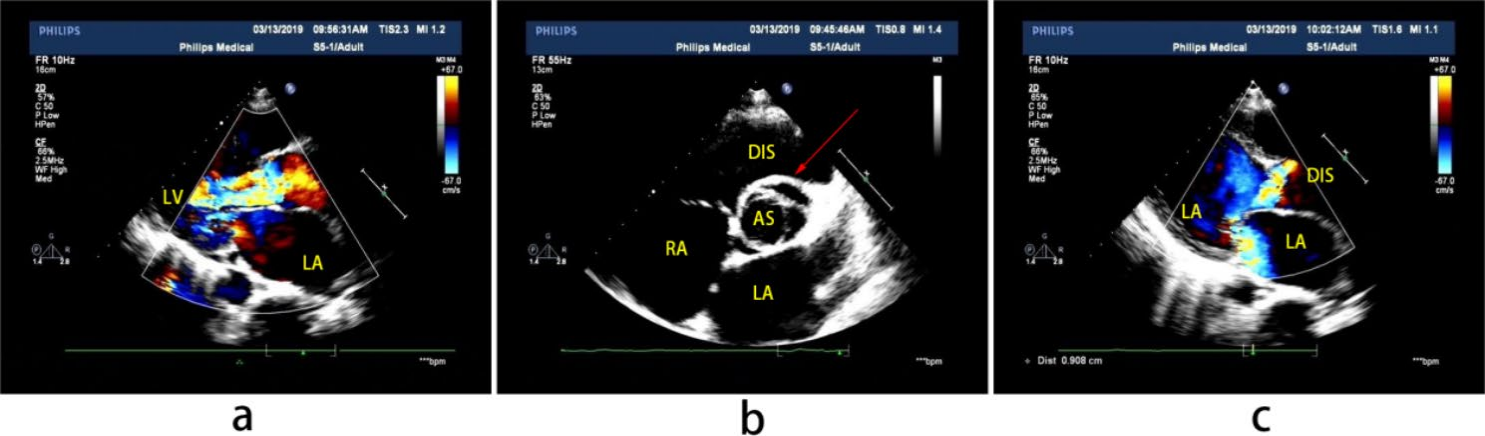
Pre-operation echocardiography of this patient. (a) Formation of interventricular septal dissection (yellow arrow), entry into left ventricle behind the anechoic area in the upper part of the interventricular septum. (b) Rupture of right aortic sinus, blood flowing from the right sinus part to the interventricular septal dissection and then to the left ventricle. (c) Mitral regurgitation. LV, left ventricle; LA, left atrium; RA, right atrium; AS, aortic sinus; DIS, dissection
